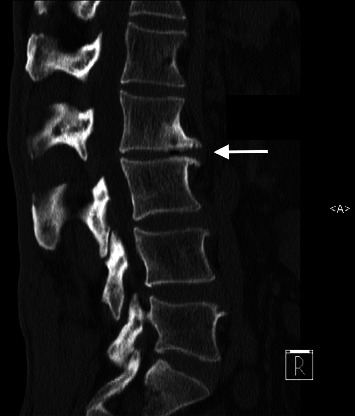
Computed tomography image of the lumbar spine shows disc space degeneration at multiple levels, predominantly at L2-3 (arrow).
